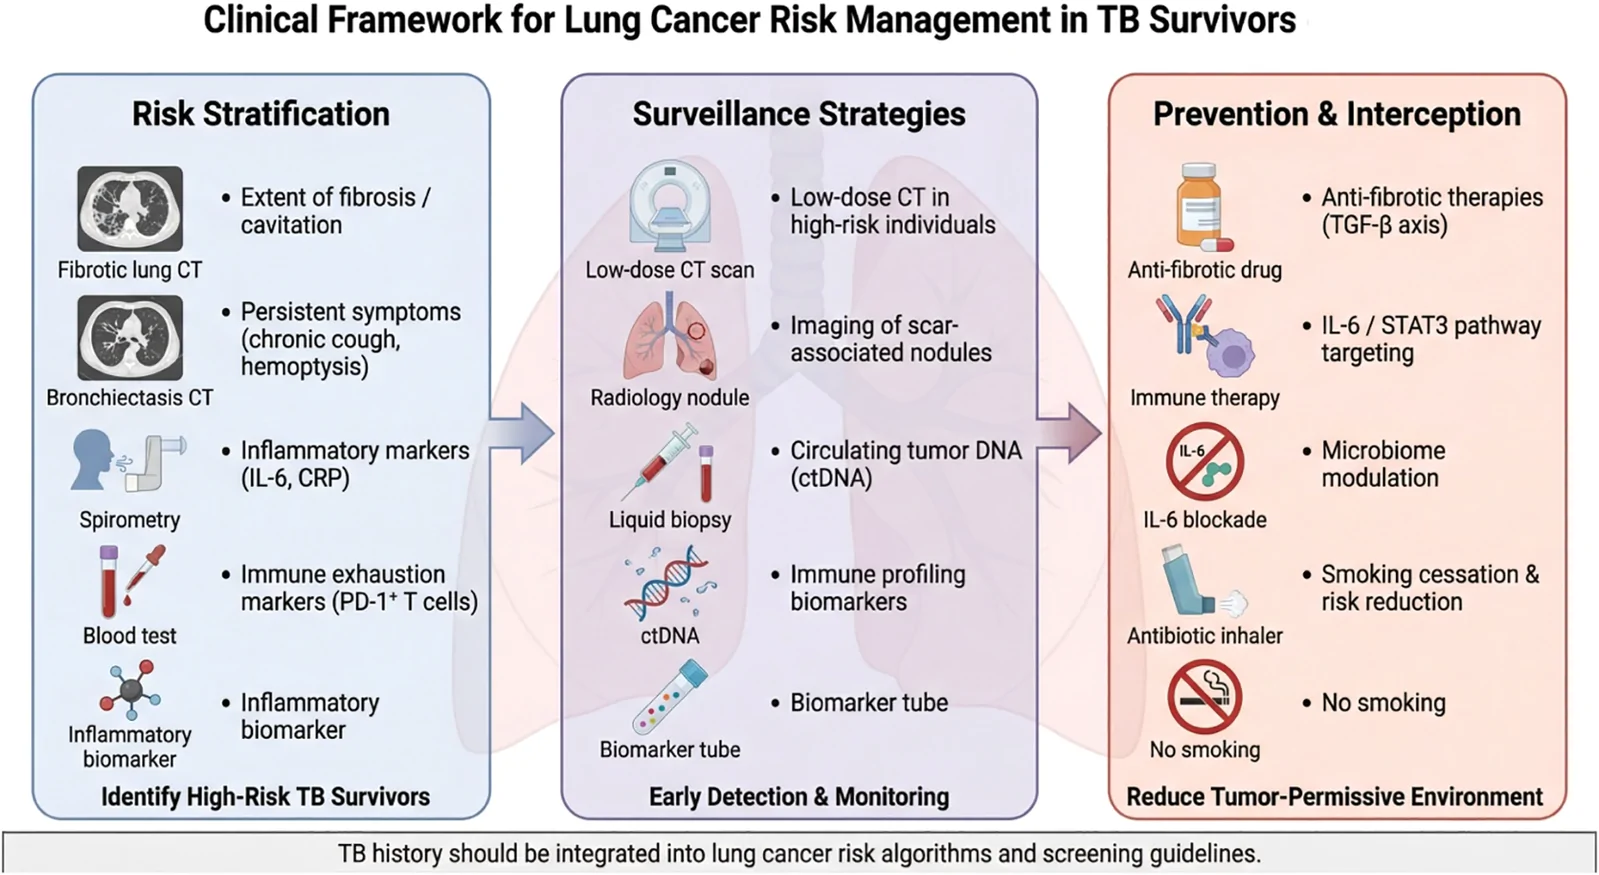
Translational framework for lung cancer risk management in tuberculosis survivors. Structural and immune features of post-TB lung disease may allow risk stratification based on fibrosis burden, persistent inflammation, and immune exhaustion markers. High-risk individuals could benefit from tailored surveillance using low-dose CT and emerging biomarkers such as circulating tumor DNA or immune profiling. Preventive strategies, including anti-fibrotic therapies, modulation of inflammatory signaling pathways, microbiome-directed interventions, and risk reduction measures, may help mitigate the tumor-permissive microenvironment. Integrating TB history into lung cancer risk algorithms may improve early detection and outcomes. Created with Biorender.com.
